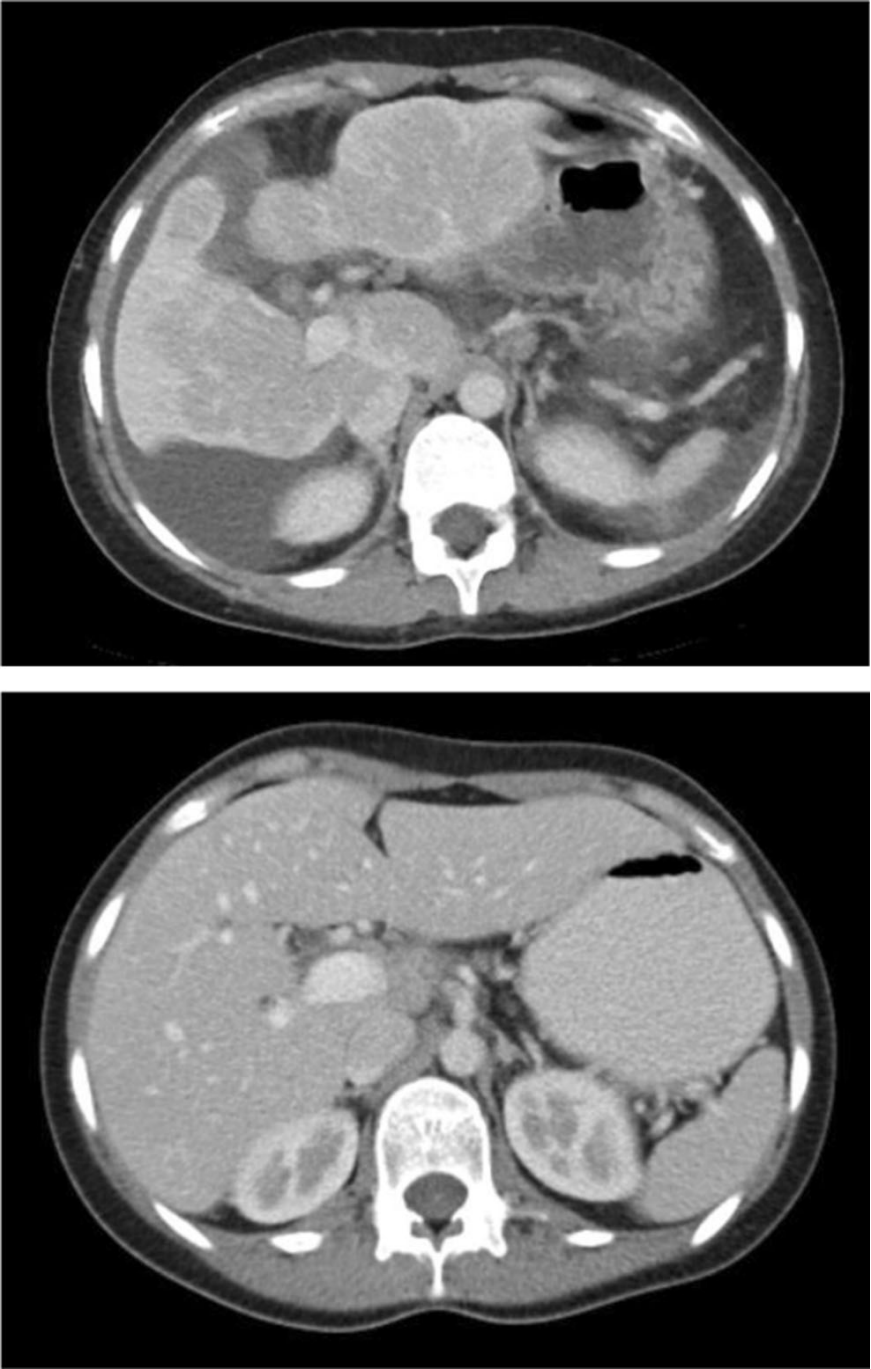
Portal venous phase CT shows multiple hypo-enhancing pseudomasses representing areas of hepatic necrosis with background of ascites. This is biopsy-proven fulminant hepatic necrosis. The necrosis-induced fibrosis and atrophy cause hepatic capsular retraction, and there has been substantial volume loss compared with a CT performed 7 months earlier (*bottom image*)
